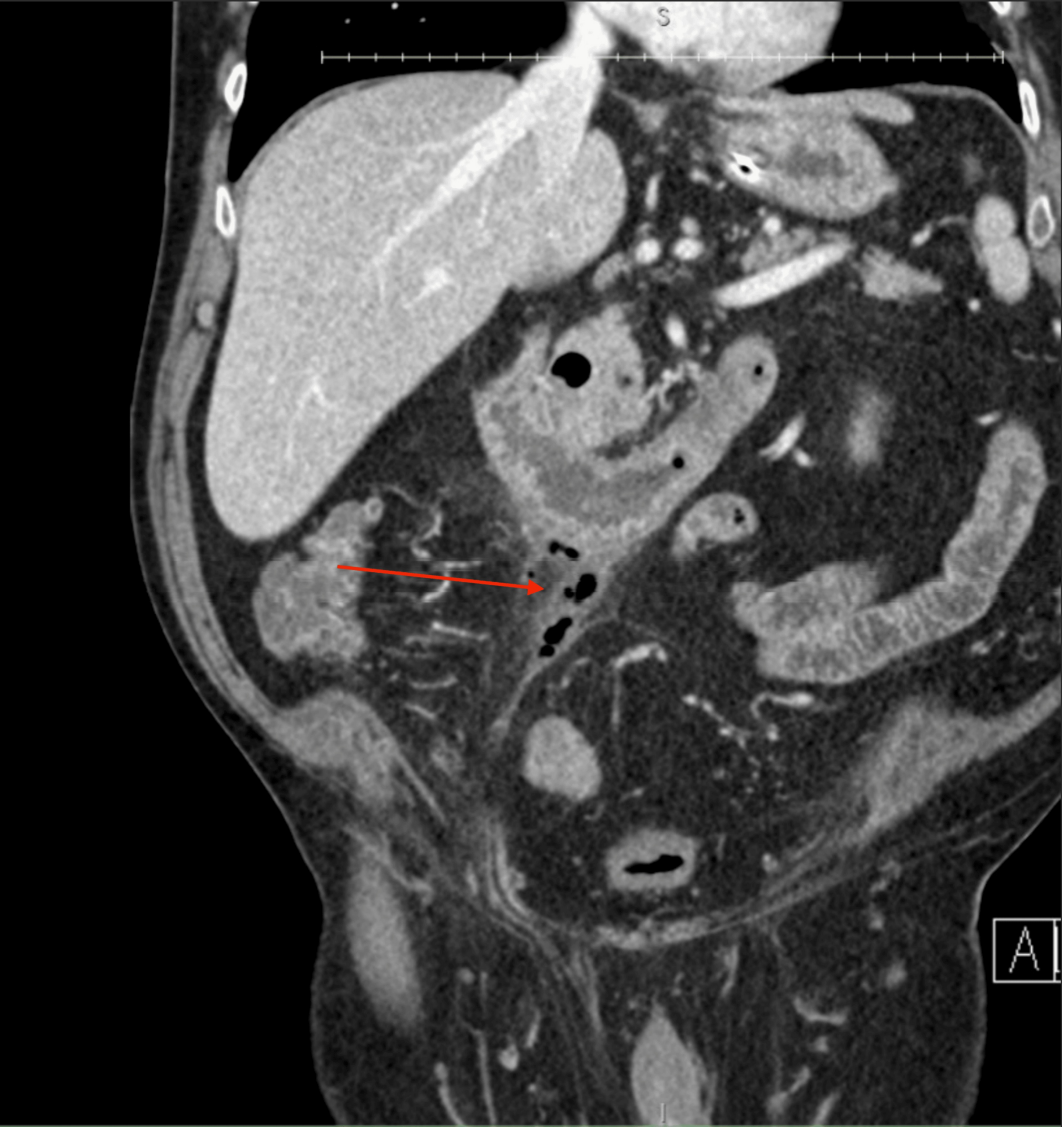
CT abdomen/pelvis demonstrating a contained perforation with hazy stranding and extraluminal gas on the anteroinferior margin of the duodenum.
